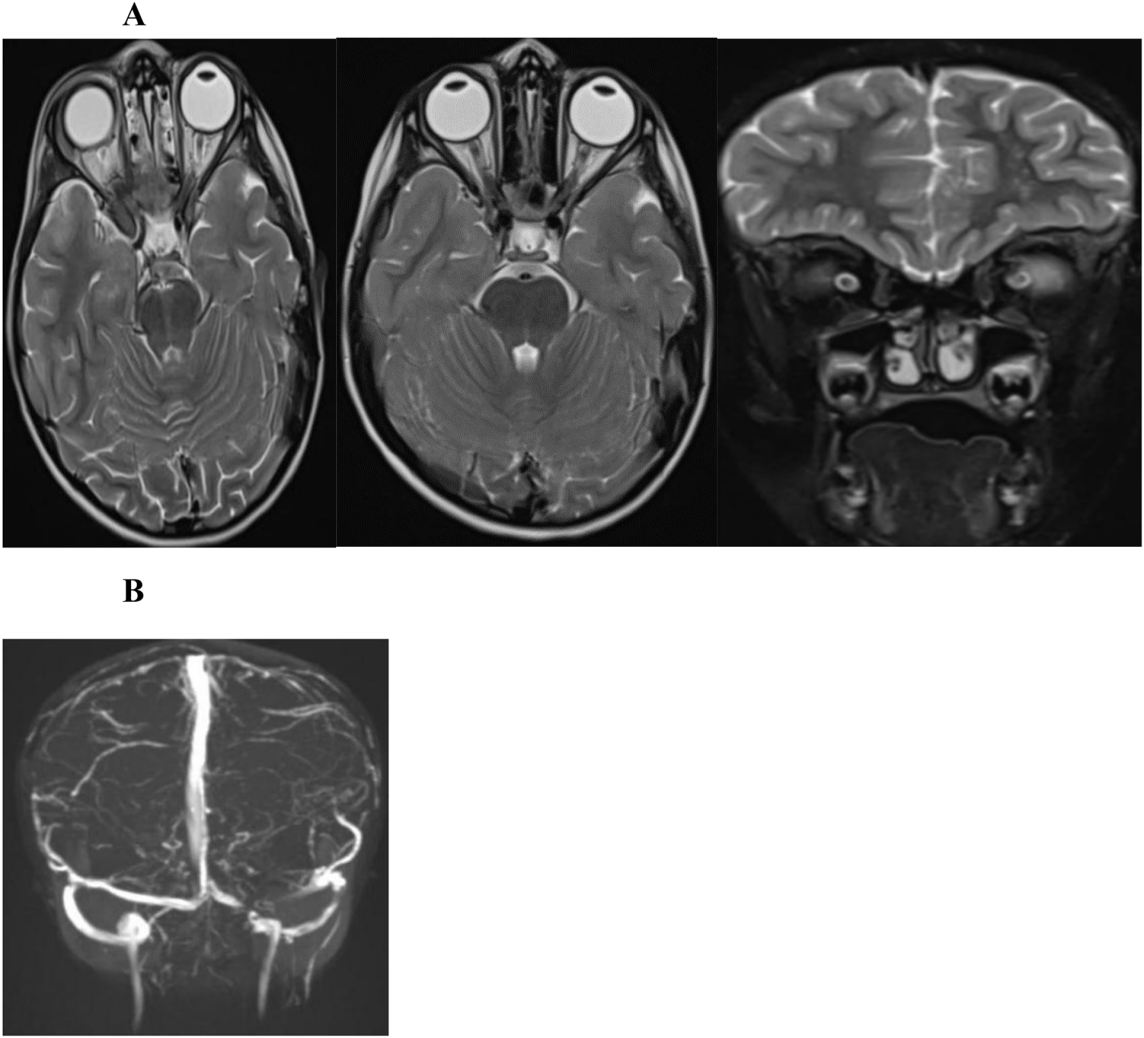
Baseline neuroimaging prior to treatment. (A) 2 Axial and one coronal T2-weighted MRI of the brain and orbits at the level of optic nerve heads, demonstrate dilatation of the optic nerve sheath complexes with tenting of the optic nerve heads and flattening of the posterior globes, consistent with elevated intracranial pressure. (B) MR venography demonstrates thrombosis of the left transverse sinus, sigmoid sinus, and jugular bulb, with non-enhancing filling defects.
